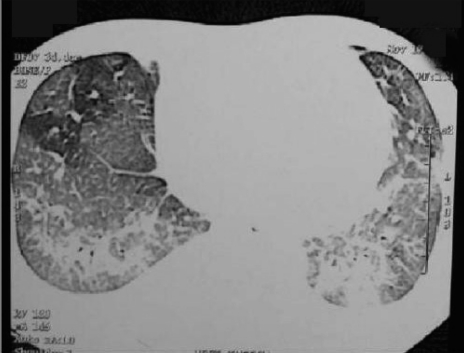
CT scan of chest of Case 2, showing bilateral basal ground glass opacities with interlobular septal thickening
